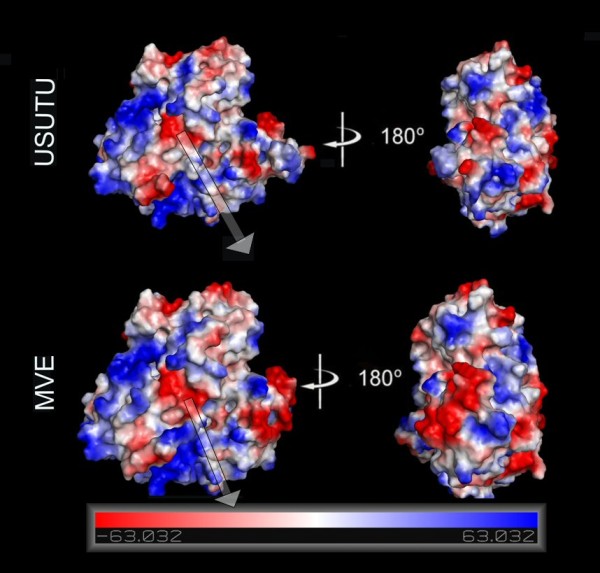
Electrostatic surfaces potential for the Usutu virus helicase model and the Murray Valley Encephalitis virus helicase template (X-ray structure: 2V80). White transparent arrow is showing the nucleic acid channel (ssRNA).
